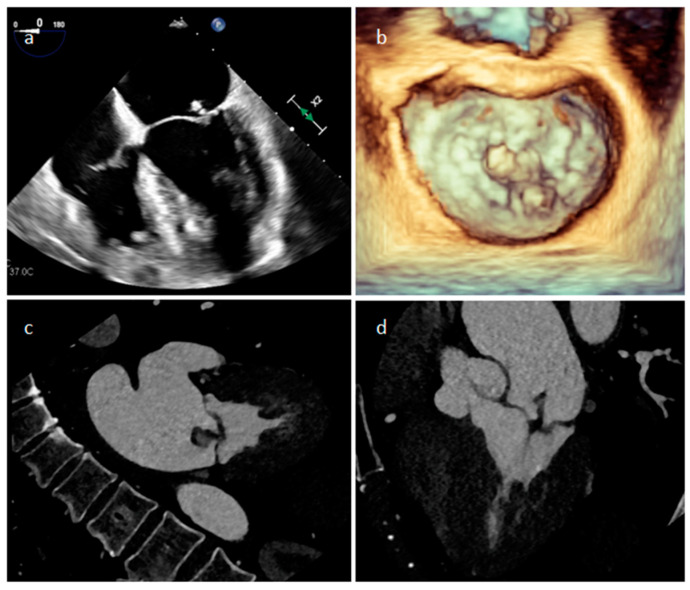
A mitral valve vegetation at 2D (a) and 3D (b) transesophageal echocardiography, and at CT (c,d).
